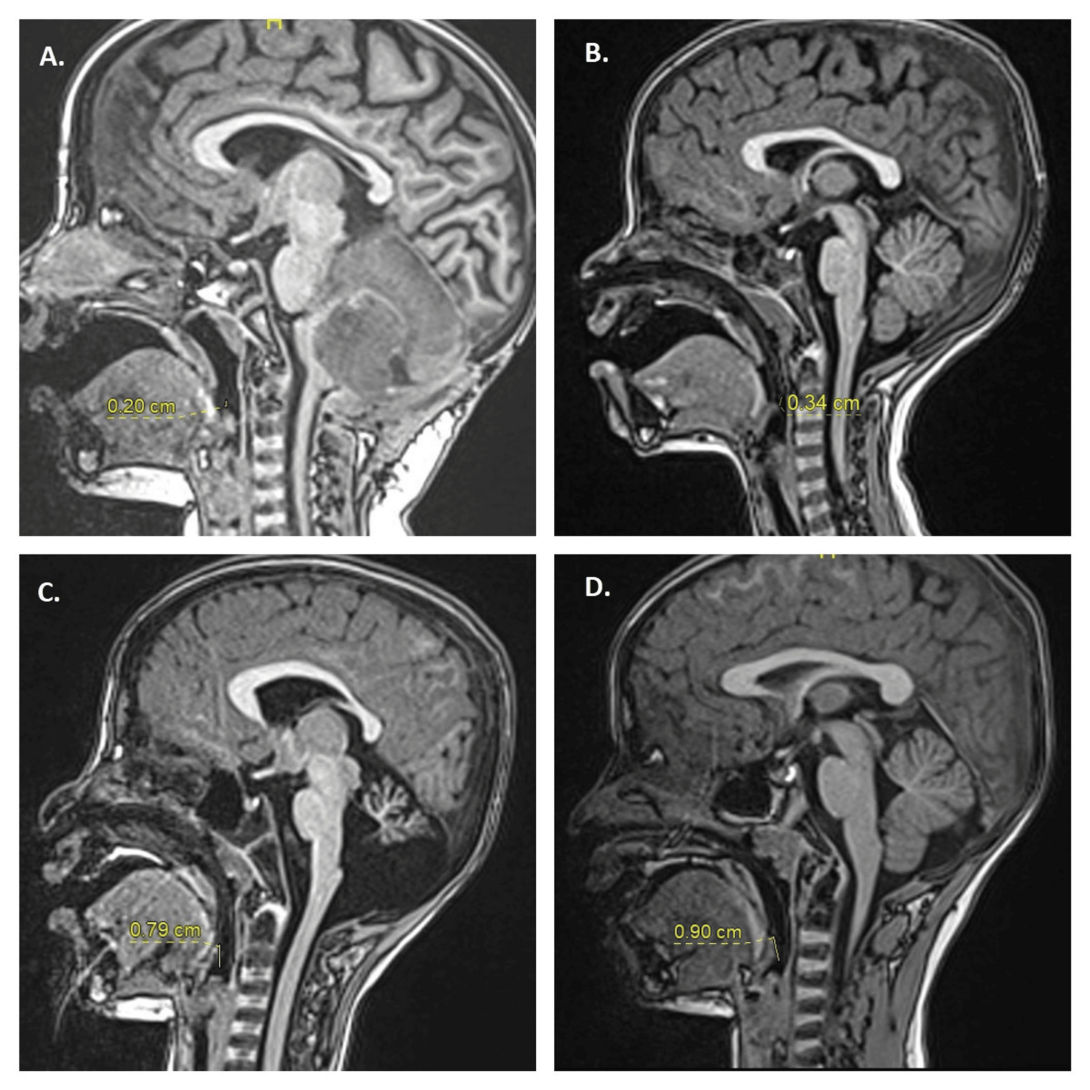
Magnetic resonance imaging (MRI) sagittal sections demonstrating the position of the nasopharyngeal airway (NPA) tip relative to the epiglottis NPA tip positioned above the epiglottis by 0.2 cm (A), above the tip of the epiglottis by 0.34 cm (B), below the tip of the epiglottis by 0.79 cm (C), and below the tip of the epiglottis by 0.9 cm (D).
